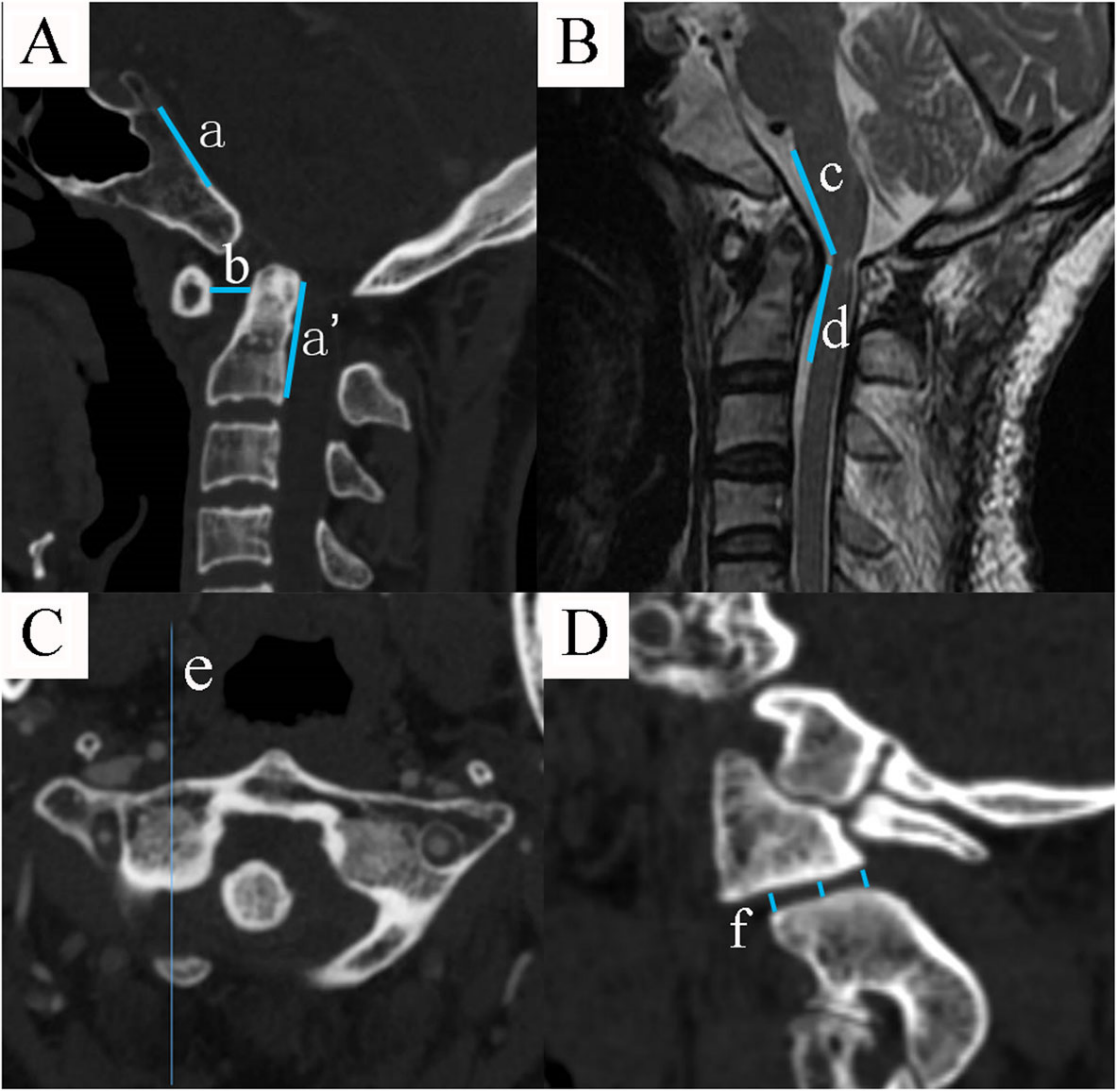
a The crossing angle of the line of inferior 1/3 clivus (line a) and the line extending from the posterior border of the dens to the posterior-inferior border of the axis body (line a’) was defined as clivus-canal angle (CCA). The distance from the posterior border of the anterior C1 arch to the anterior border of the odontoid process (line b) was measured as anterior atlantodental interval (ADI). b The crossing angle of the line extending the anterior border of the ventral medulla (line c) and the line extending the anterior border of the ventral upper cervical spinal cord (line d) in midline sagittal T2 MRI was defined as cervicomedullary angle (CMA). c and d The middle line of the lateral mass in the cross-sectional slice was defined as line e. The corresponding sagittal reconstruction CT based on line e was seen in D. We measured the anterior part, middle part, and posterior part of the lateral mass interspace, and the average value was defined as the lateral mass interspace (LMI). The combined bilateral LMI was defined as sum of LMI (SLMI)
